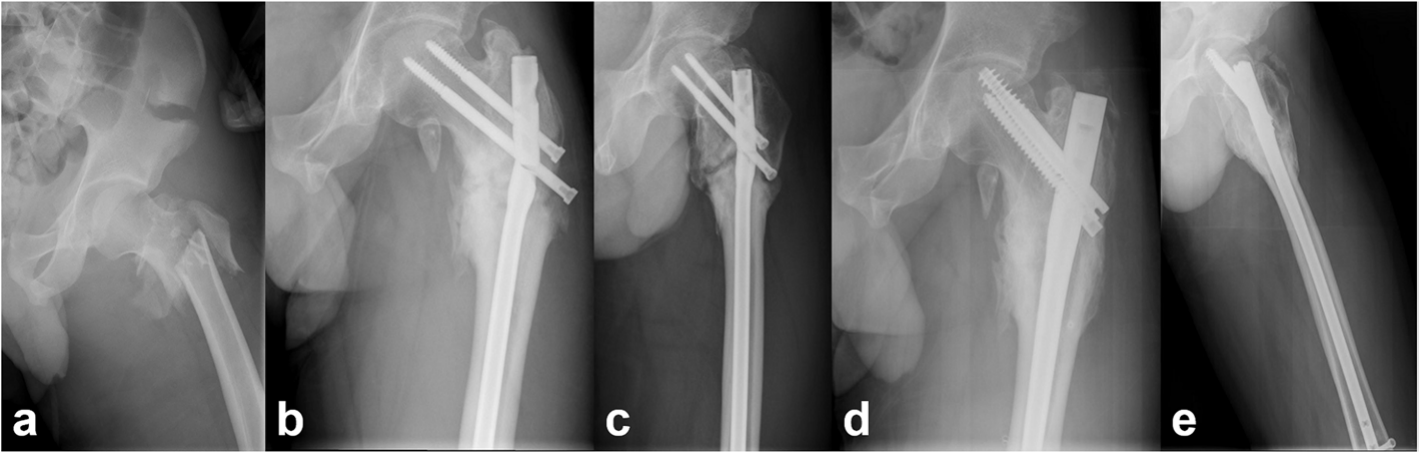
A 23-year-old man with left trochanteric fracture (AO/OTA A3) was initially fixed by a long femoral reconstruction intramedullary nail. a The X-ray of original fracture. b-c The initial implant broke ten months after the operation with the fractur nonunion. d-e The previous implant was replaced with an InterTAN without medial support reconstruction, and the fracture healed 6 months after revision surgery
